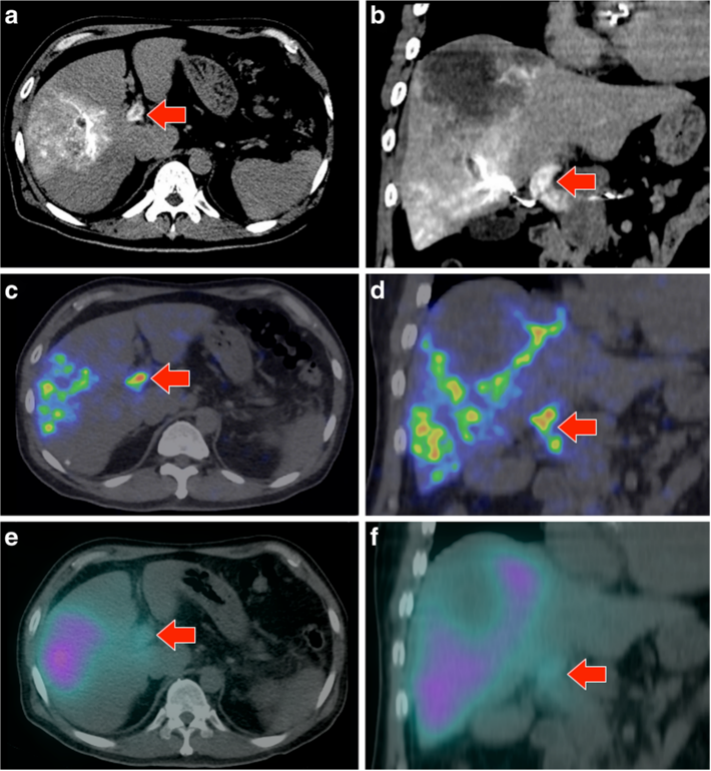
Patient 10. Portal vein tumor thrombosis in a large heterogeneous HCC. (a, b) Catheter-directed CT angiogram of the right hepatic artery demonstrates contrast enhancement within portal vein tumor thrombosis (arrow). (c, d)^90^Y PET/CT depicts focal ^90^Y activity within the portal vein tumor thrombus in high resolution. (e, f) The same activity is poorly visualized on ^90^Y bremsstrahlung SPECT/CT as subtle, ill-defined activity.
